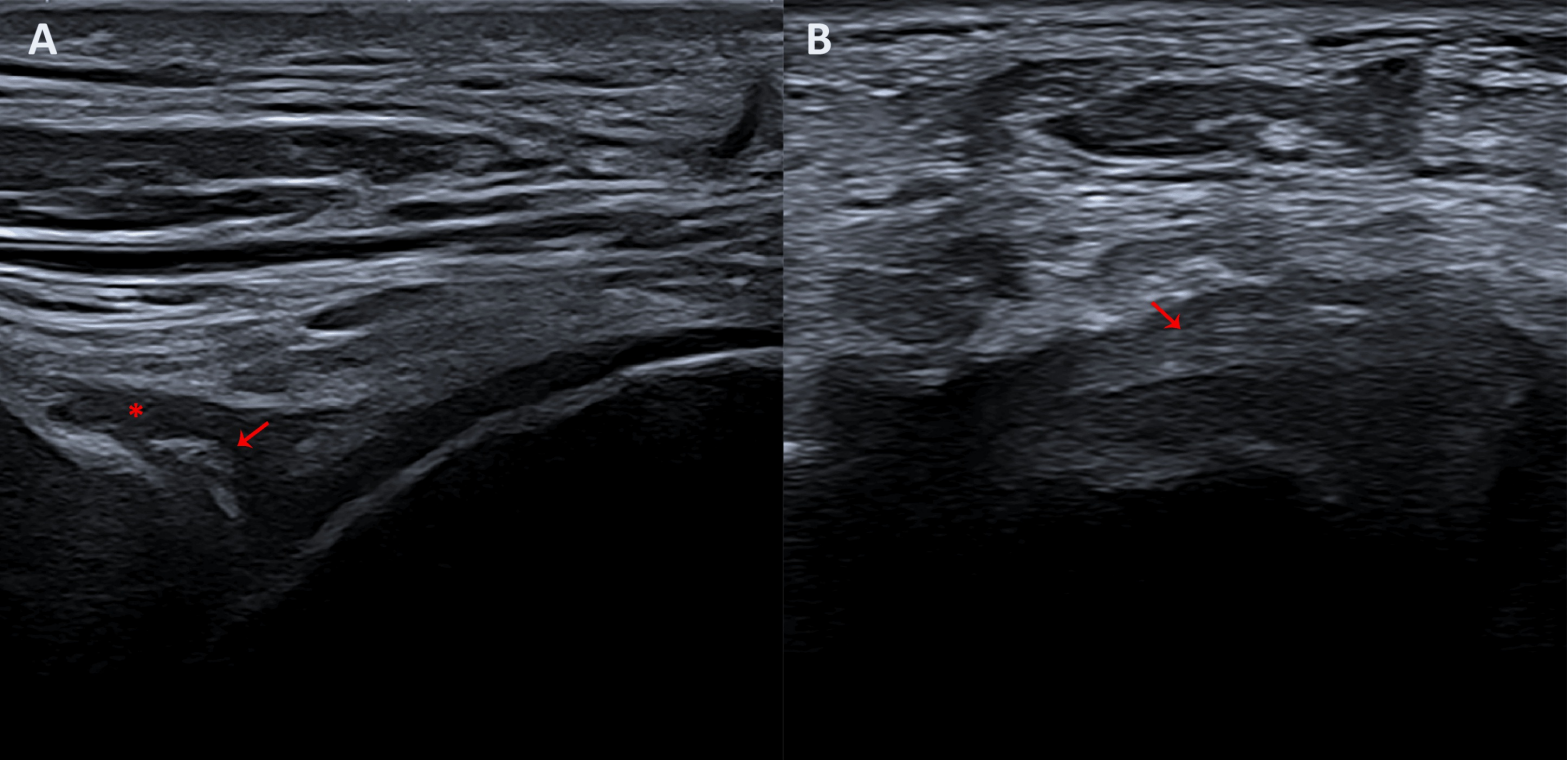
Ultrasound images focused on the anterior talocrural recess, confirming persistent intra-articular findings. (A) Longitudinal view demonstrating persistent joint effusion (red asterisk) and an intra-articular fibrous band traversing the recess (red arrow). (B) Axial view confirming the presence of the fibrous band (red arrow) within the anterior recess.
